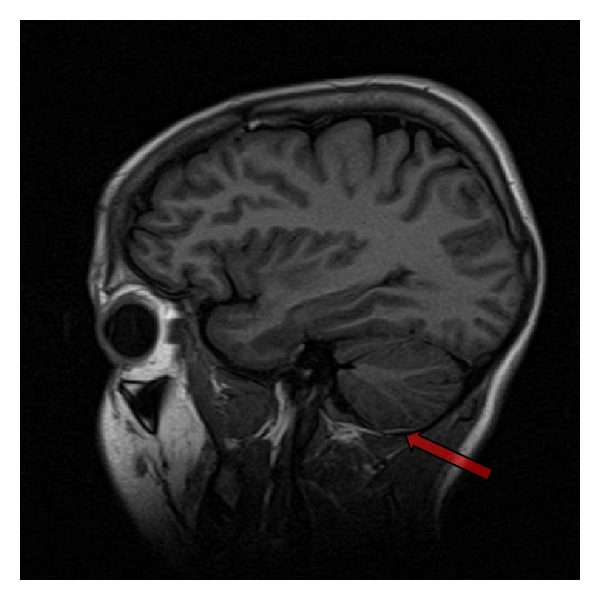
Sagittal T1-weighted image of the brain demonstrates a very thin mildly hyperintense subdural hematoma over the left cerebellar convexity.
